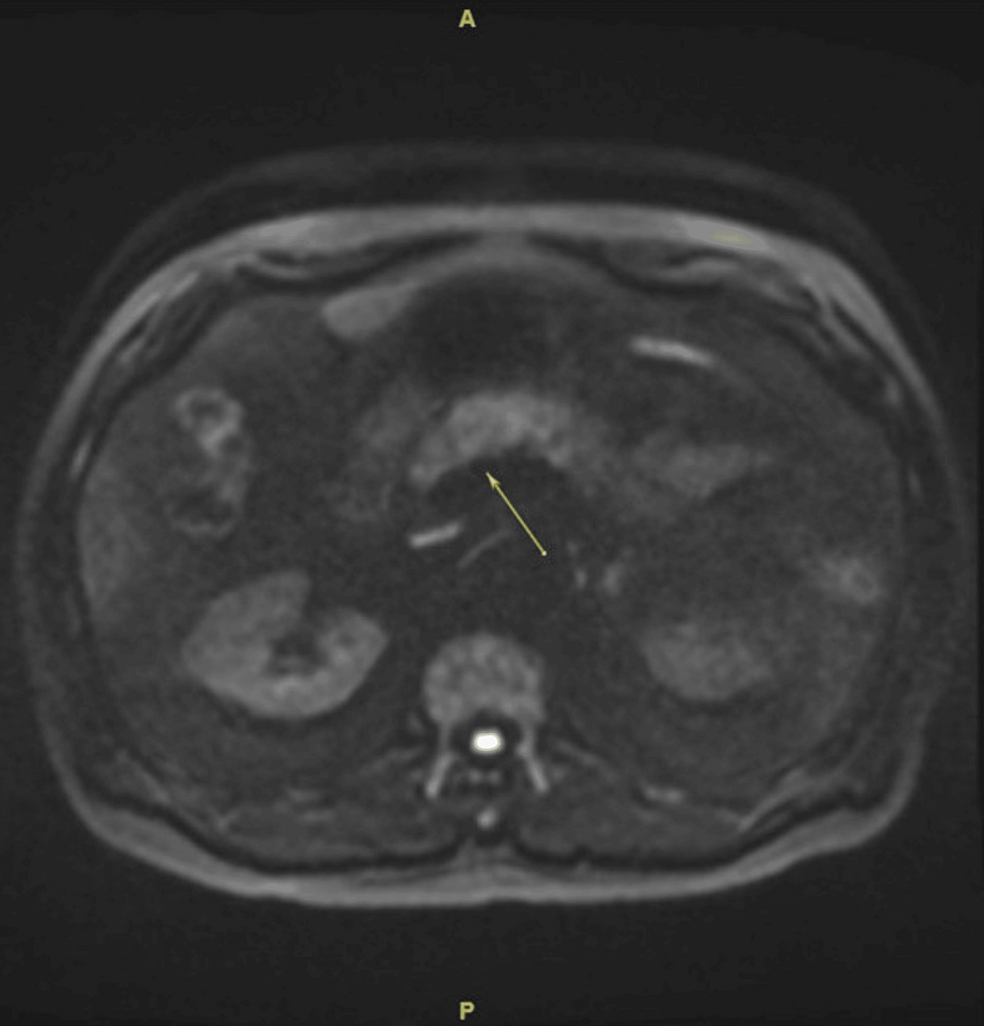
MRCP image from the first episode of pancreatitis From the first episode of pancreatitis, Figure 1 is an MRCP image that shows a mildly increased DWI signal within the pancreatic head and body (arrow). This is radiographic evidence of acute pancreatitis given the patient's clinical presentation. MRCP: Magnetic resonance cholangiopancreatography; DWI: Diffusion-weighted imaging
